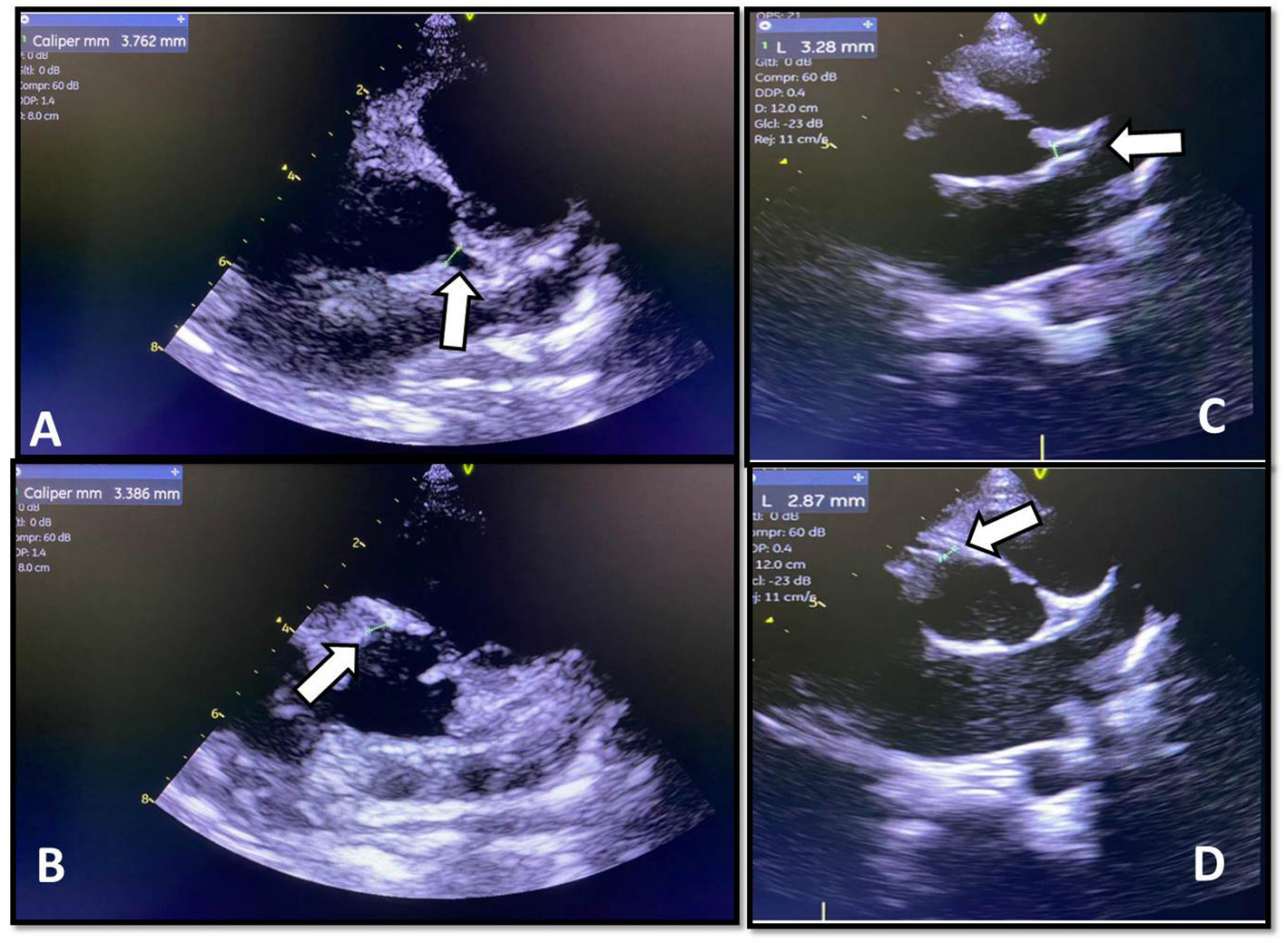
(A) Pretreatment echocardiographic images of the left main coronary artery measuring 3.762 mm (*Z* score +3.22). (B) Pretreatment echocardiographic images of the proximal right coronary measuring 3.38 mm (*Z* score +2.86). (C) Posttreatment echocardiographic images of the left main coronary artery measuring 3.28 mm (*Z* score +2.04). (D) Posttreatment echocardiographic images of the right proximal coronary artery measuring 2.87 mm (*Z* score +1.66).
